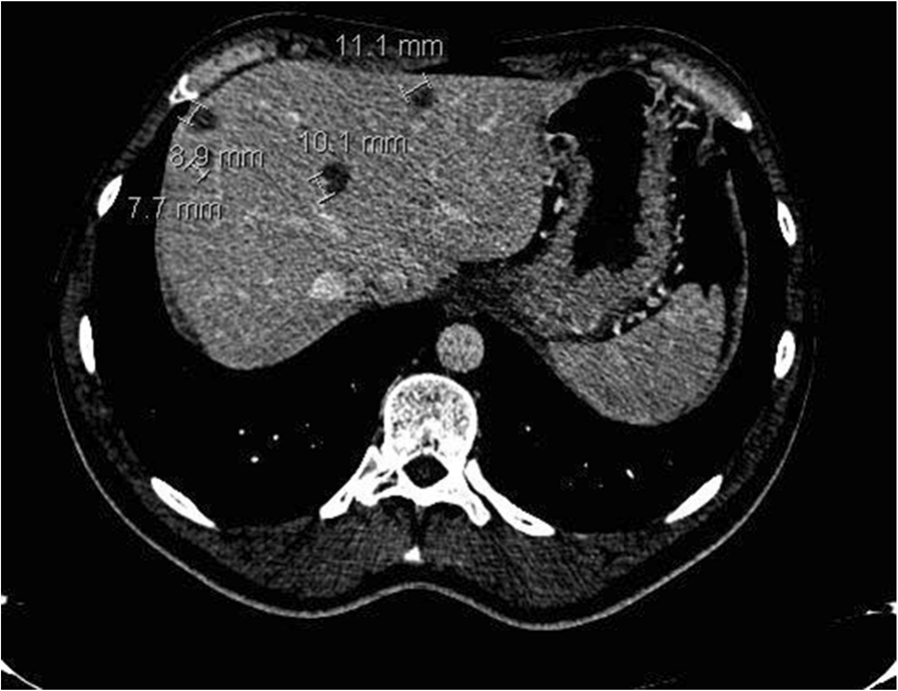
Abdominal CT-scan fifteen months after end of chemotherapy with further regression of pulmonary fibrosis, and regression of liver lesions.
